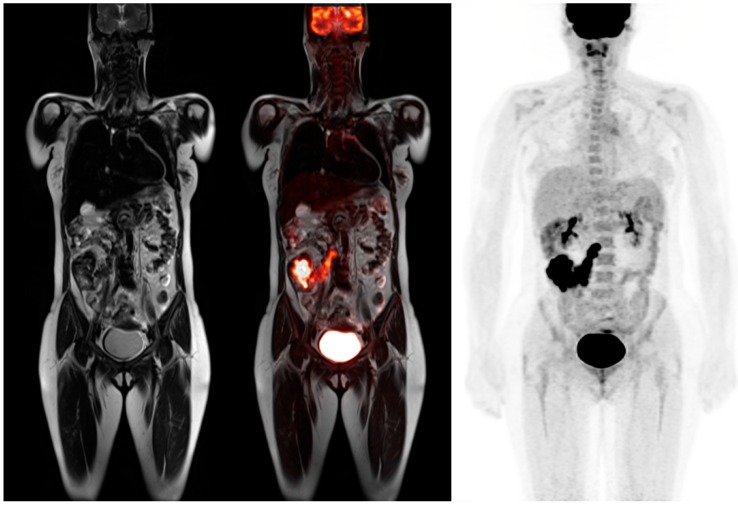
45-year-old female for initial staging of colon cancer. T2 coronal image shows a large right colon mass with multiple low signal intensity lymph nodes (*left*). PET-MRI coronal fusion images better reveal the colon mass and chain of mesenteric lymph nodes that extends to the paracaval region (*middle*). PET MIP image shows the prominent FDG activity in the colon cancer and lymph node metastases (*right*).
